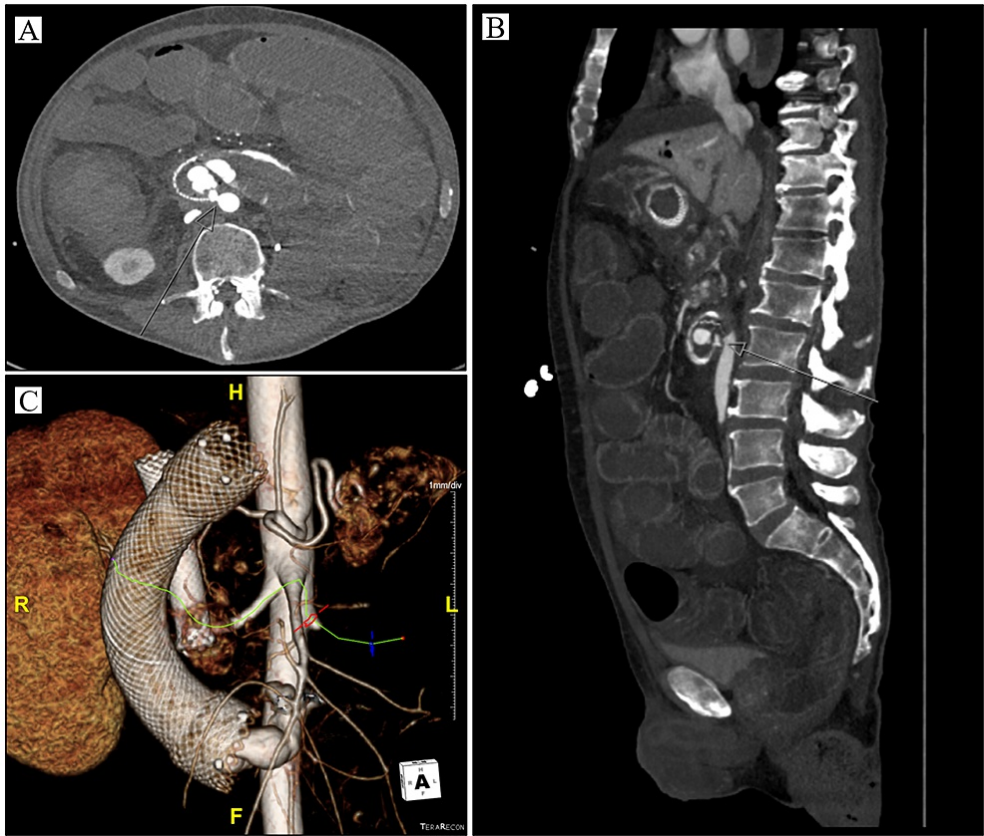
Abdominal imaging following stabilization. CT of the abdomen and pelvis with IV contrast visualizing AEF in the transverse plane (A), sagittal plane (B), and with 3D reconstruction (C). AEF, aortoenteric fistula
